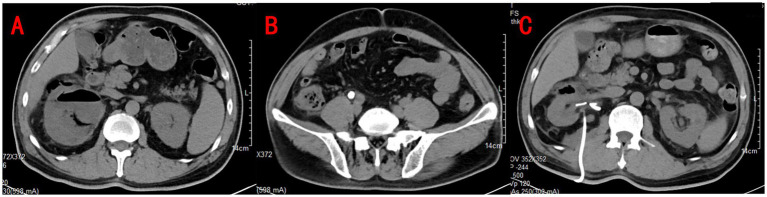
Urinary CT revealed gas in the right renal parenchyma and perirenal with right hydronephrosis (A) and right ureteral calculi at the intersection with the iliac vessels (B). Repeat CT showed a significant improvement in right hydronephrosis and pyelonephritis with reduced gas accumulation (C).
